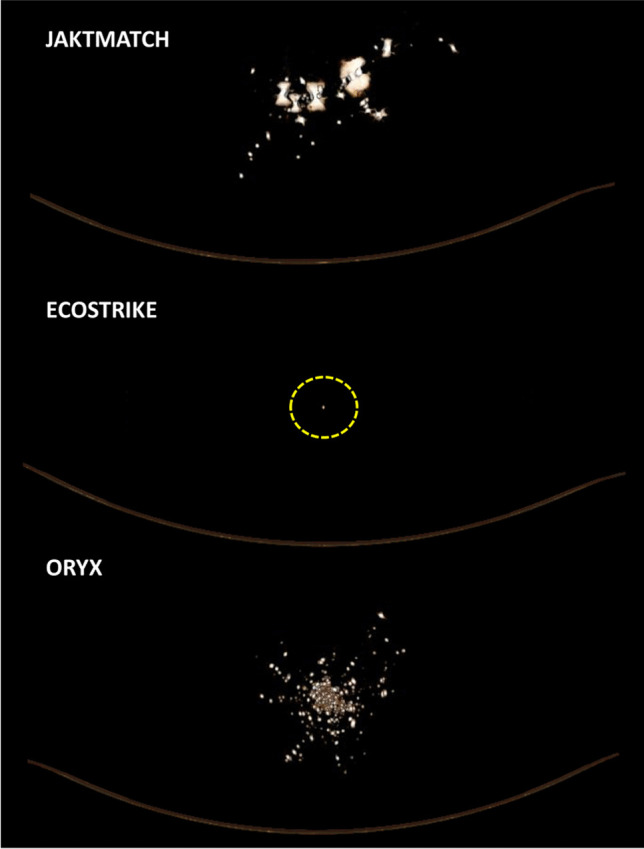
Perpendicular view of three-dimensional bullet fragment reconstruction based on computed tomography of the gelatine blocks. Bullet direction was away from the viewer. Dashed circle is used to demarkate the only fragment identified for Ecostrike
